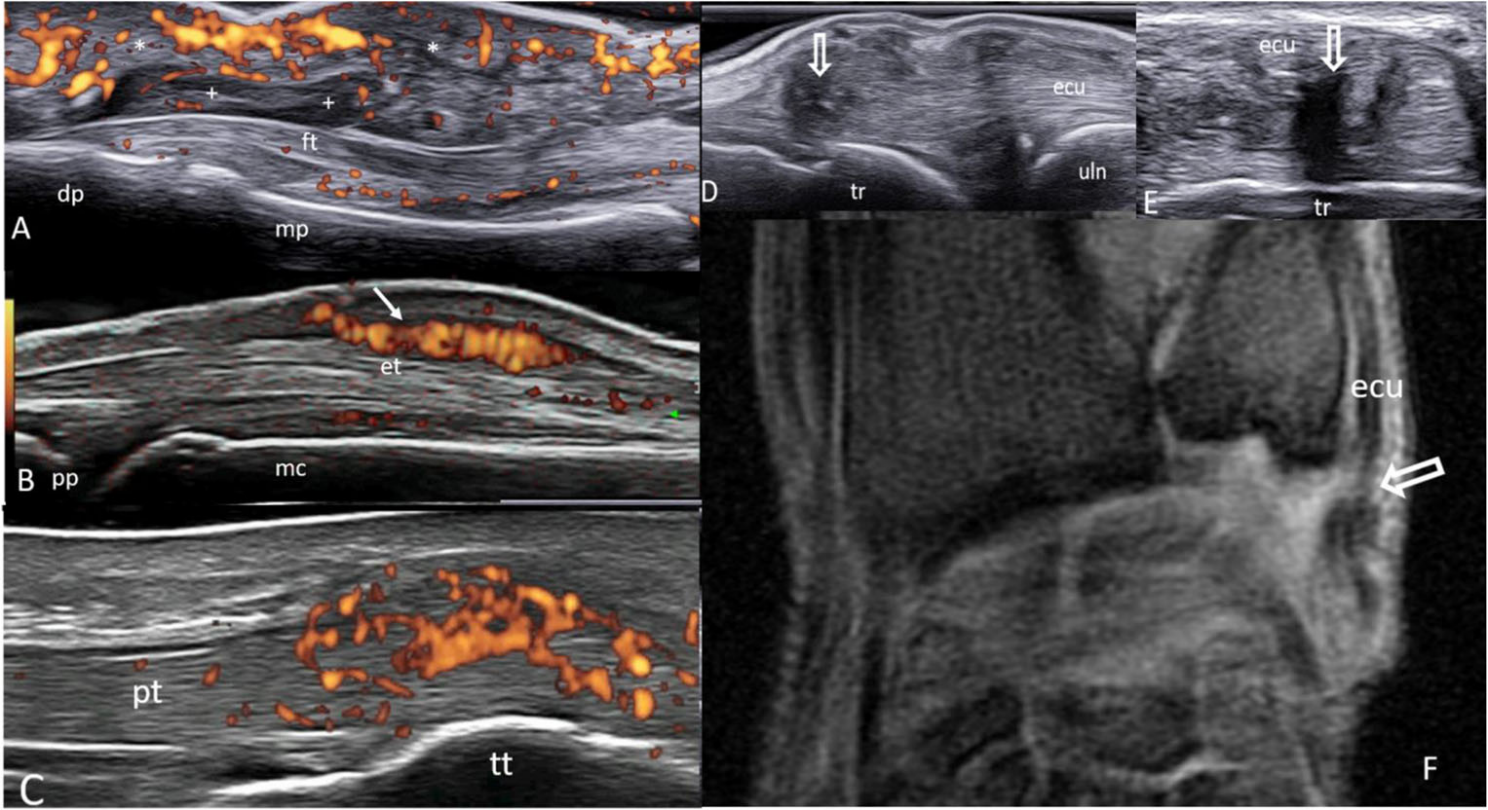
Tendon involvement in SLE. A Longitudinal volar ultrasound (US) scan of a 3rd finger of the hand obtained with a 8–24 MHz probe showing ‘active’ tenosynovitis (synovial hypertrophy within the tendon sheath: +) of the flexor digitorum profundus tendon. Note the presence of marked soft tissue edema (asterisks). B Longitudinal dorsal US scan of a 3rd metacarpophalangeal joint obtained with a 6–18 MHz probe showing peritendinitis (arrow) of the finger extensor tendon. C Longitudinal US scan of the distal insertion of a patellar tendon obtained with a 6–18 MHz probe depicting ‘active’ enthesitis, characterized by entheseal thickening and intense power Doppler signal (red spots). Intra-tendinous tear (open arrow) of an extensor carpi ulnaris tendon by US (D, longitudinal scan; E, transverse scan) and corresponding magnetic resonance imaging (F). dp = distal phalanx; ecu = extensor carpi ulnaris; et = finger extensor tendon; ft = flexor digitorum profondus tendon; mc = metacarpal bone; mp = middle phalanx; pp = proximal phalanx; pt = patellar tendon; tr = triquetrum. tt = tibial tuberosity; uln = ulnar styloid
